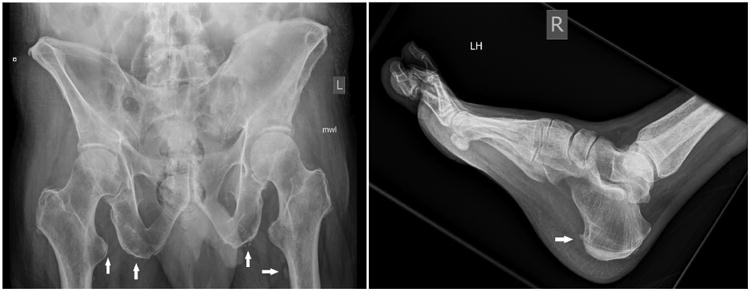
X-rays from Patient 2 (hip left panel; ankle and foot right panel). Films show enthesopathy involving multiple pelvic regions as well as the right heel. White arrows indicate ligamentous ossification characteristic of enthesopathy. Bone eburnation was also noted.
